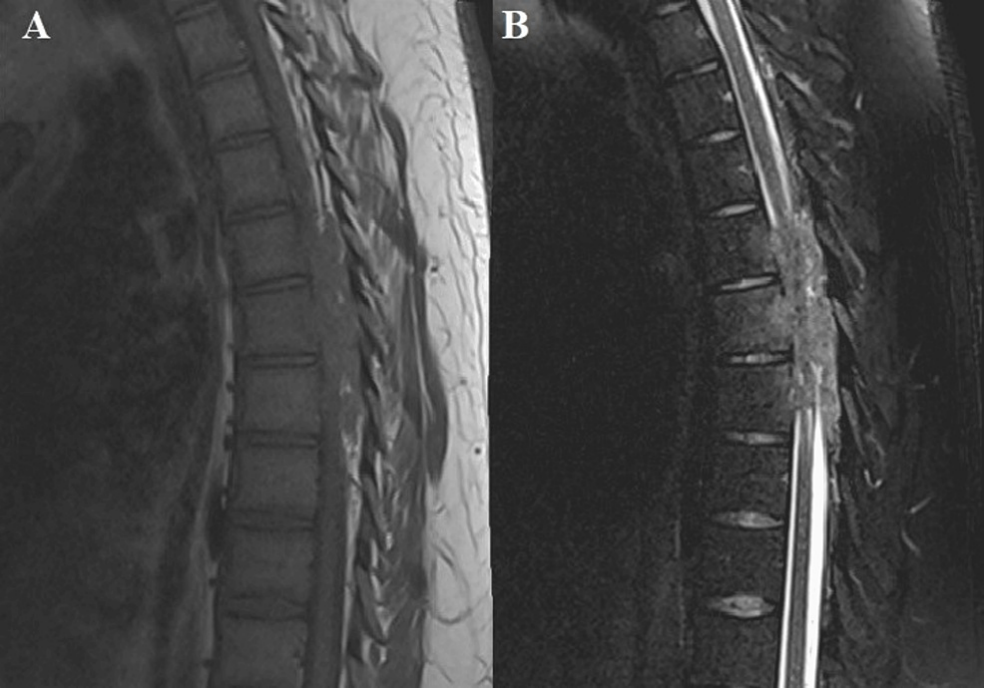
(A and B). MRI of the thoracic spine in T1 and T2 sequences in sagittal projections showing Ewing's sarcoma located from TH5 to TH7 vertebral projections and its relationship to the adjacent dura, as well as medullary compression. In Figure 6A on T1 sequence, the tumor has a hypointense density. In Figure 6B, Ewing's sarcoma is presented on a hyperintense T2 sequence.
